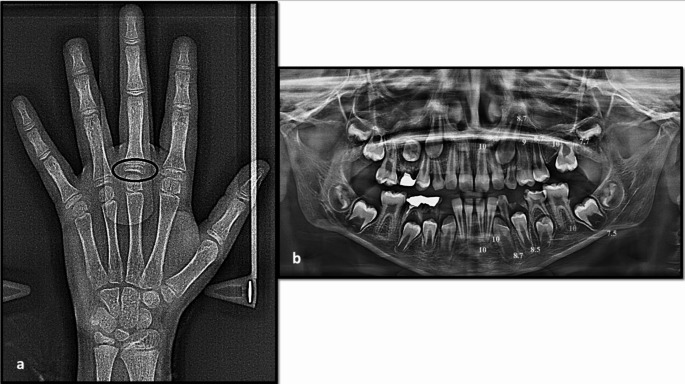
a) Hand-wrist X-ray of a 9.05-year female patient showing different sites (in black) of skeletal maturity indicators (SMI) assessed by Fishman method; the proximal phalanx of the third finger showed equal width of the epiphysis and diaphysis (SMI = 1), and skeletal age is 10.23 years, b) Panoramic X-ray of the same patient showing different scoring of dental development of the mandibular and maxillary teeth on the left side (except the third molar), marked according to the Nolla method; the total scoring was 118 and the calculated dental age was 9 years
